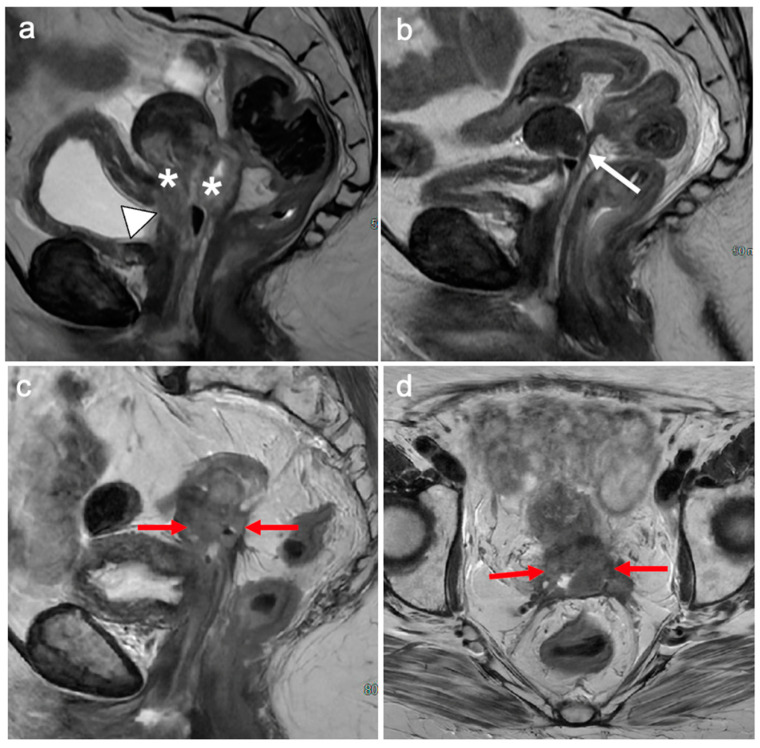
Sagittal T2WI at initial staging (a) demonstrates a bulky cervical tumour (*) with bladder involvement (arrow head). 6 month post-treatment (b) demonstrates reconstitution of the low signal cervical stroma indicating a complete response. Thin low signal intensity bands extend to the rectum consistent with post treatment fibrosis (white arrow). One year later the patient began experiencing bowel symptoms and subsequent MRI in sagittal (c) and axial (d) planes demonstrates intermediate signal intensity at the cervix (red arrows), extending to and involving the rectum consistent with recurrence.
